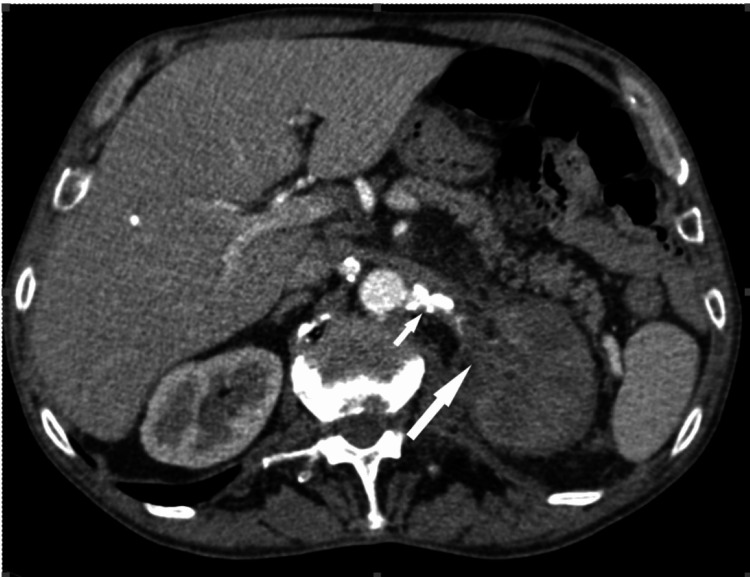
The contrast-enhanced CT (CECT) scan conducted during the arterial phase in the axial plane reveals a kidney devoid of distinguishable facial features, a characteristic manifestation attributed to transitional cell carcinoma (TCC) with infiltration into the sinus fat. Furthermore, notable within the imaging and indicated by the small arrow is the identification of renal artery stenosis, a condition induced by calcifications. This figure is the original work of the authors. Patient consent for the use of the image was obtained, as mentioned in the patient consent form (point no. 12).
